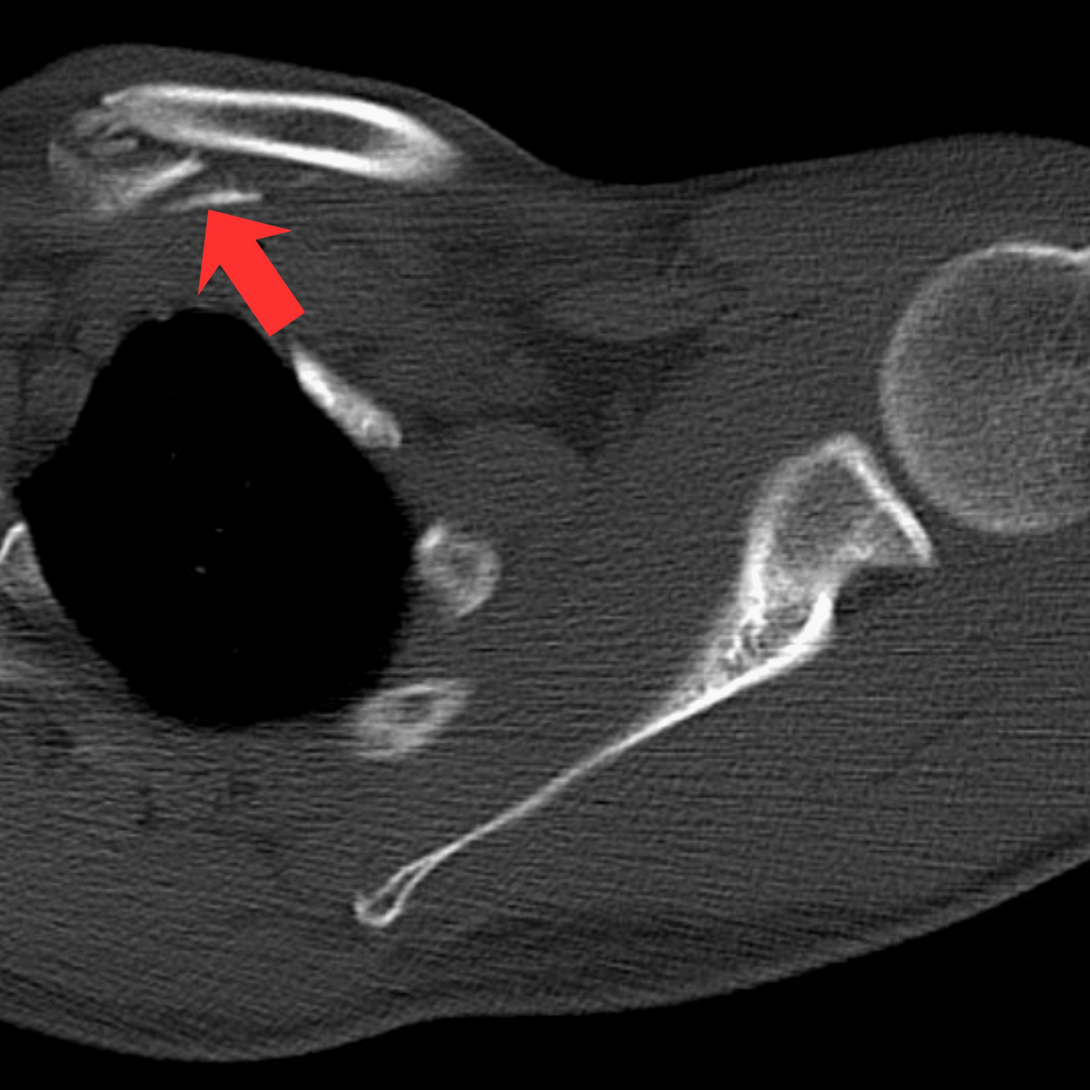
The image shows an axial view of CT scan thorax after failed sternoclavicular joint procedures. Red arrow shows persistent anteriorly subluxated sternoclavicular joint.
